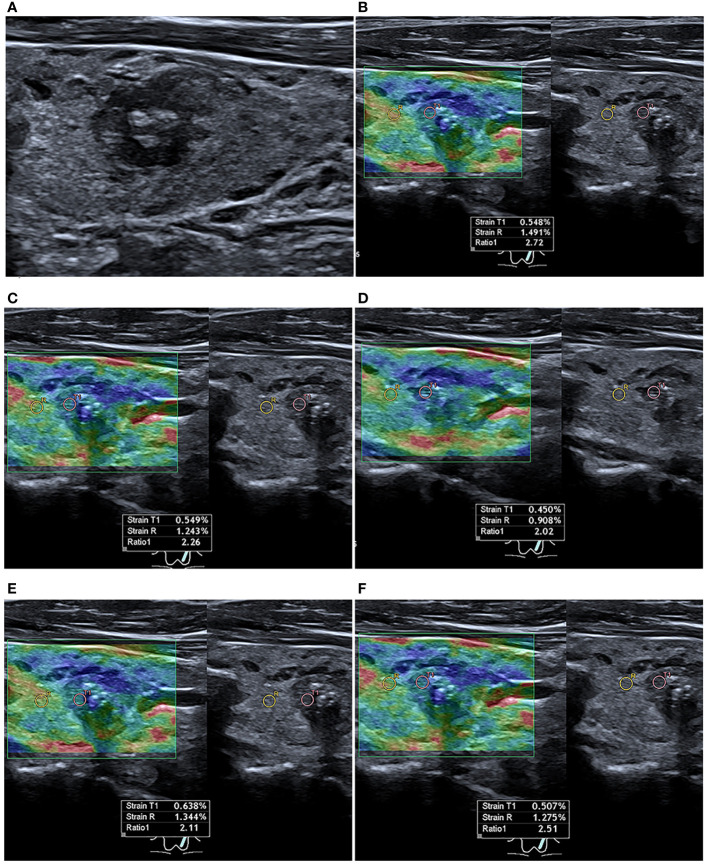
Conventional and strain ultrasound elastography of a 51-year-old male PTC patient with occult central cervical lymph node metastasis. (A) Conventional ultrasound shows a hypoechoic nodule in the lower left lobe of the thyroid gland with a maximum diameter of 12.30 mm. (B–F) Five strain ultrasound elastography images were obtained, with strain ratios of 2.72, 2.26, 2.02, 2.11, and 2.51, respectively.
